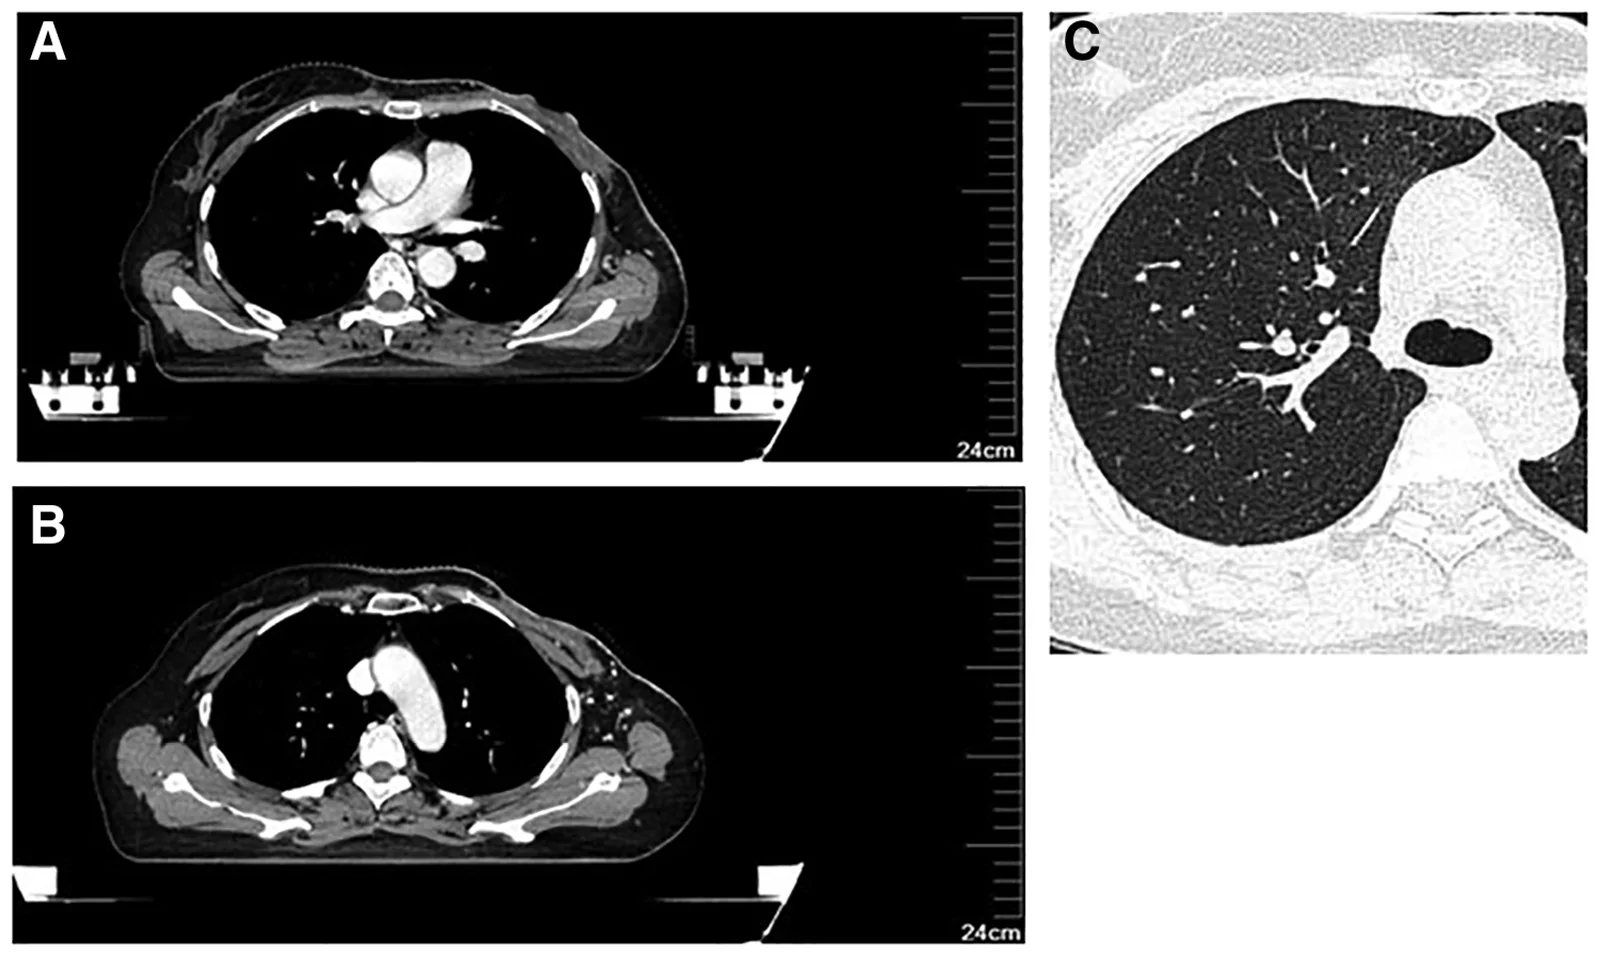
(A) Contrast-enhanced CT revealed marked regression of the (A) tumor; (B) tumor at the aortic arch level; (C) tumor at the lung. CT = computed tomography.
